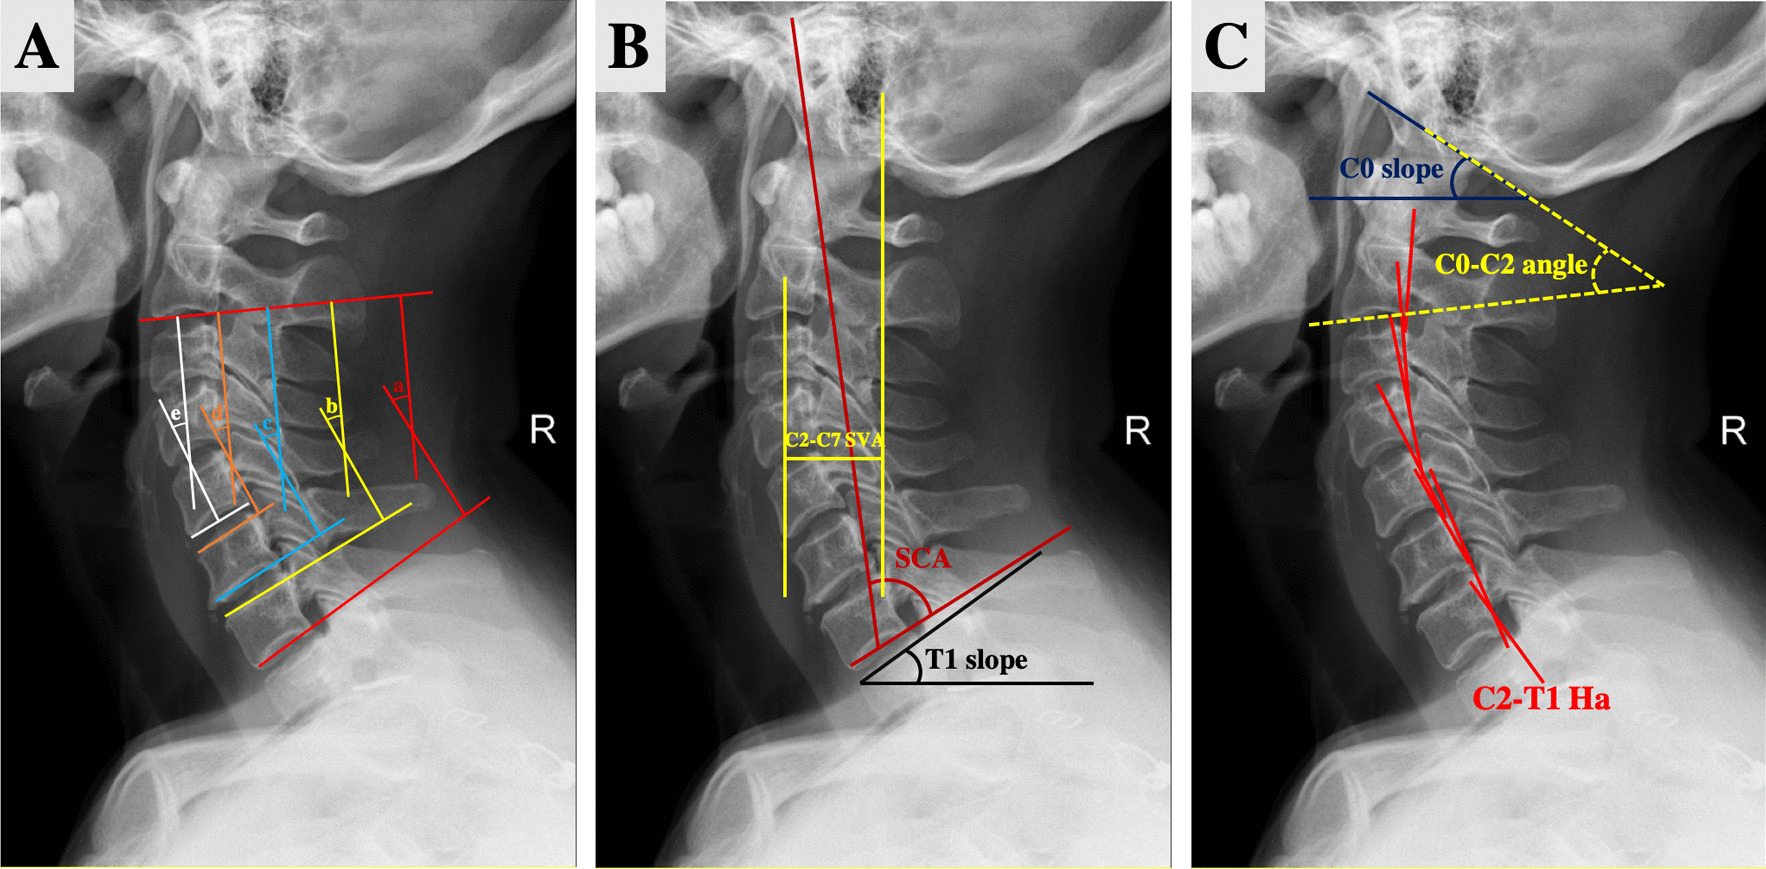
Radiographic evaluation of sagittal alignment of cervical spine. A
